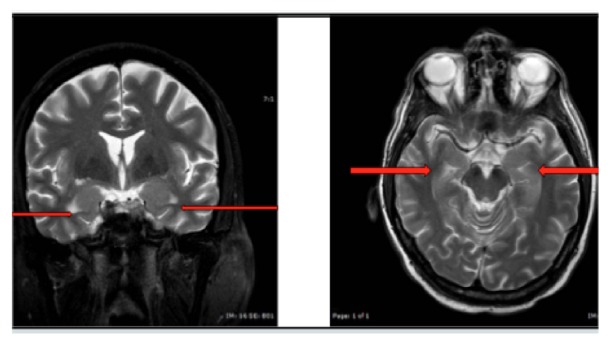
MRI brain demonstrating bilateral mesial temporal lobe hyperintensities (red arrows), right greater than left, without volume loss: (left panel) T2-weighted Coronal section; (right panel) T2-weighted with FLAIR axial section.
